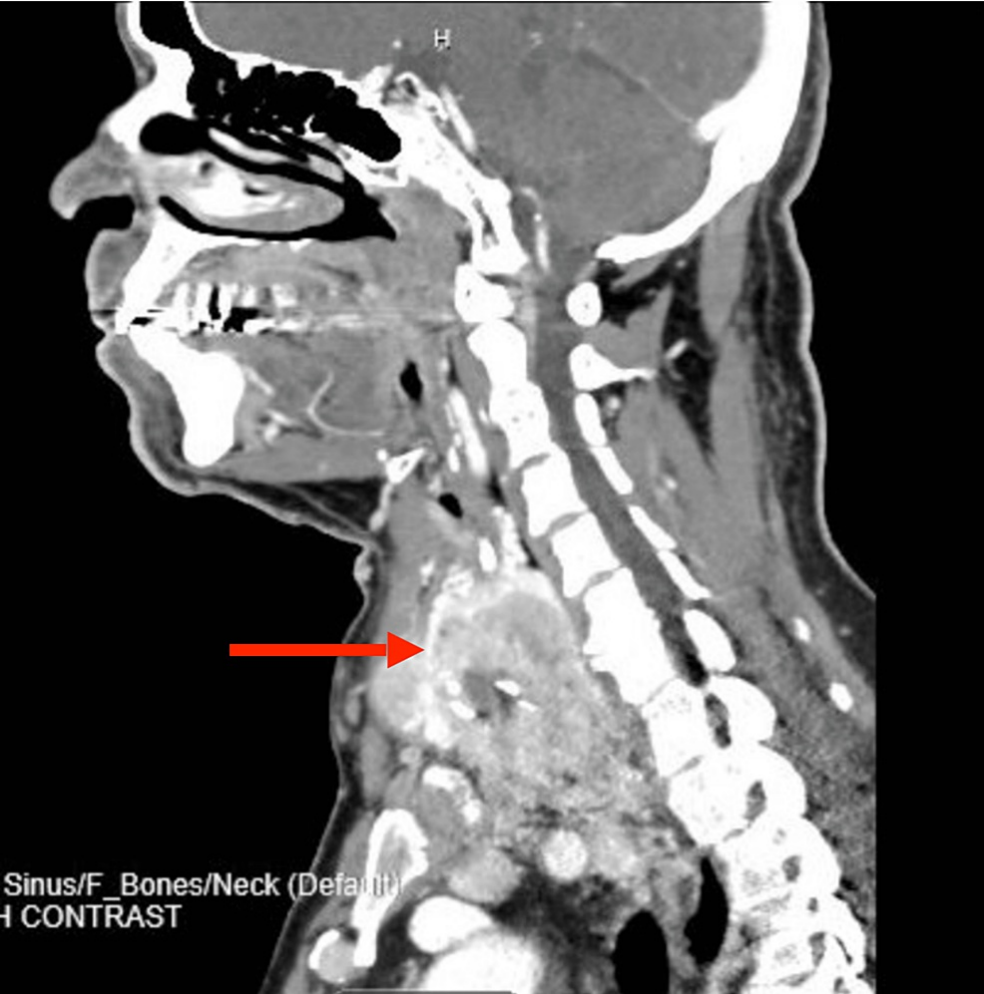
CT neck showing thyroid mass
